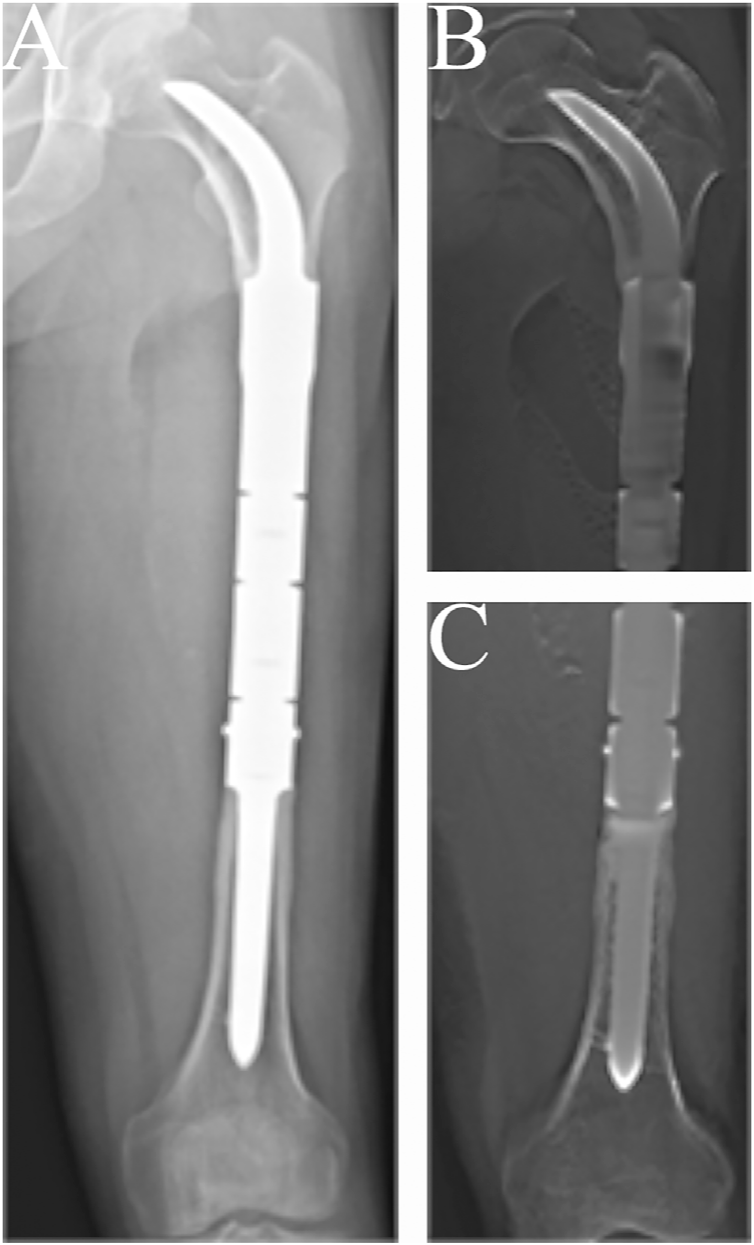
Radiographs showing the 57-months postoperative views of the customized cementless intercalary endoprosthesis with an intra-neck curved stem placed during treatment for an osteosarcoma. (A) Posteroanterior radiograph of the entire femur. (B) Posteroanterior tomosynthesis with Shimadzu Metal Artefact Reduction Technology (T-smart) views of the stem insertion region in the proximal femur. (C) Posteroanterior T-smart views of the stem insertion region in the distal femur.
